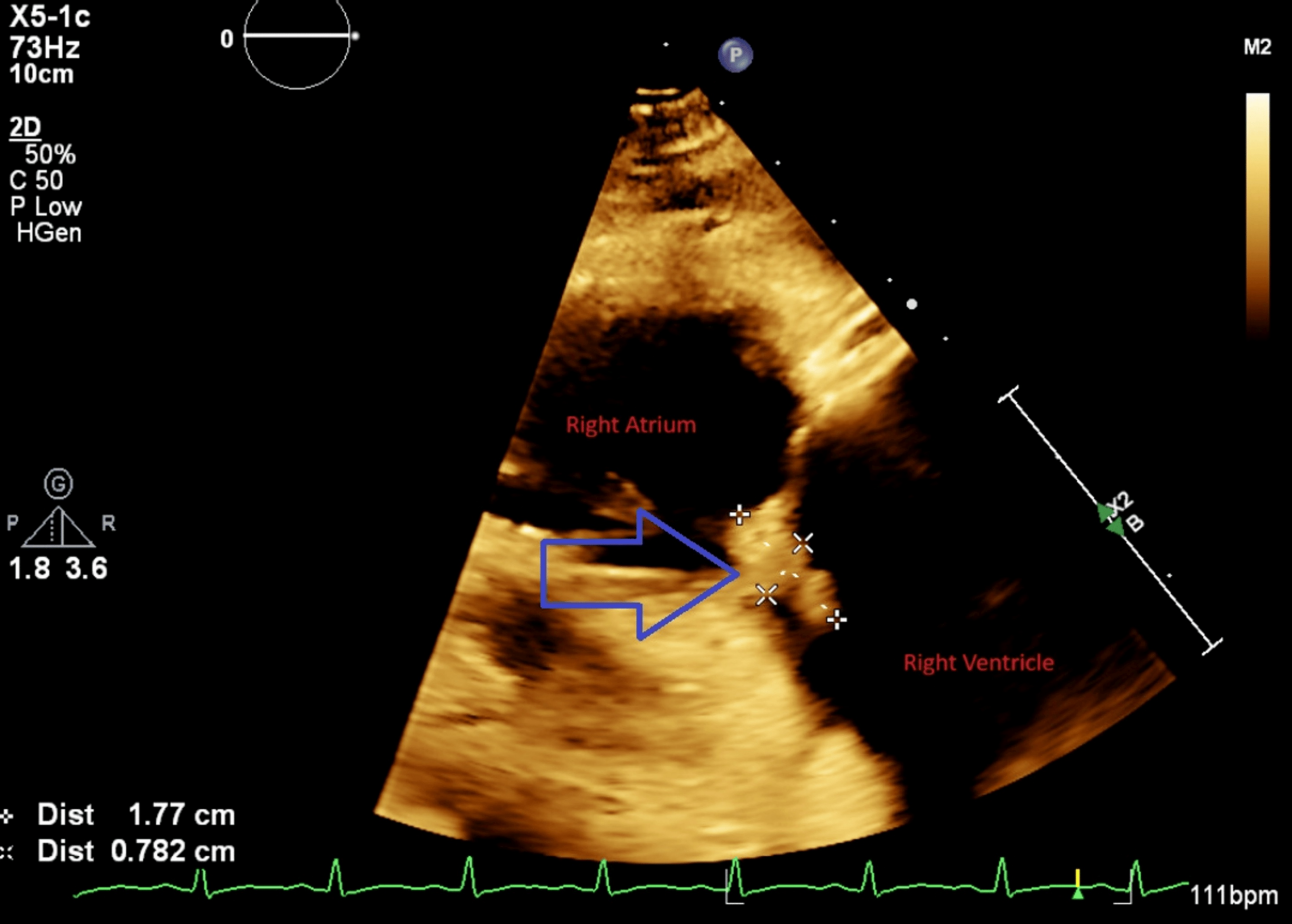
Transesophageal echocardiogram before AngioVac Blue arrow showing tricuspid vegetations (surrounded by white crosses)
